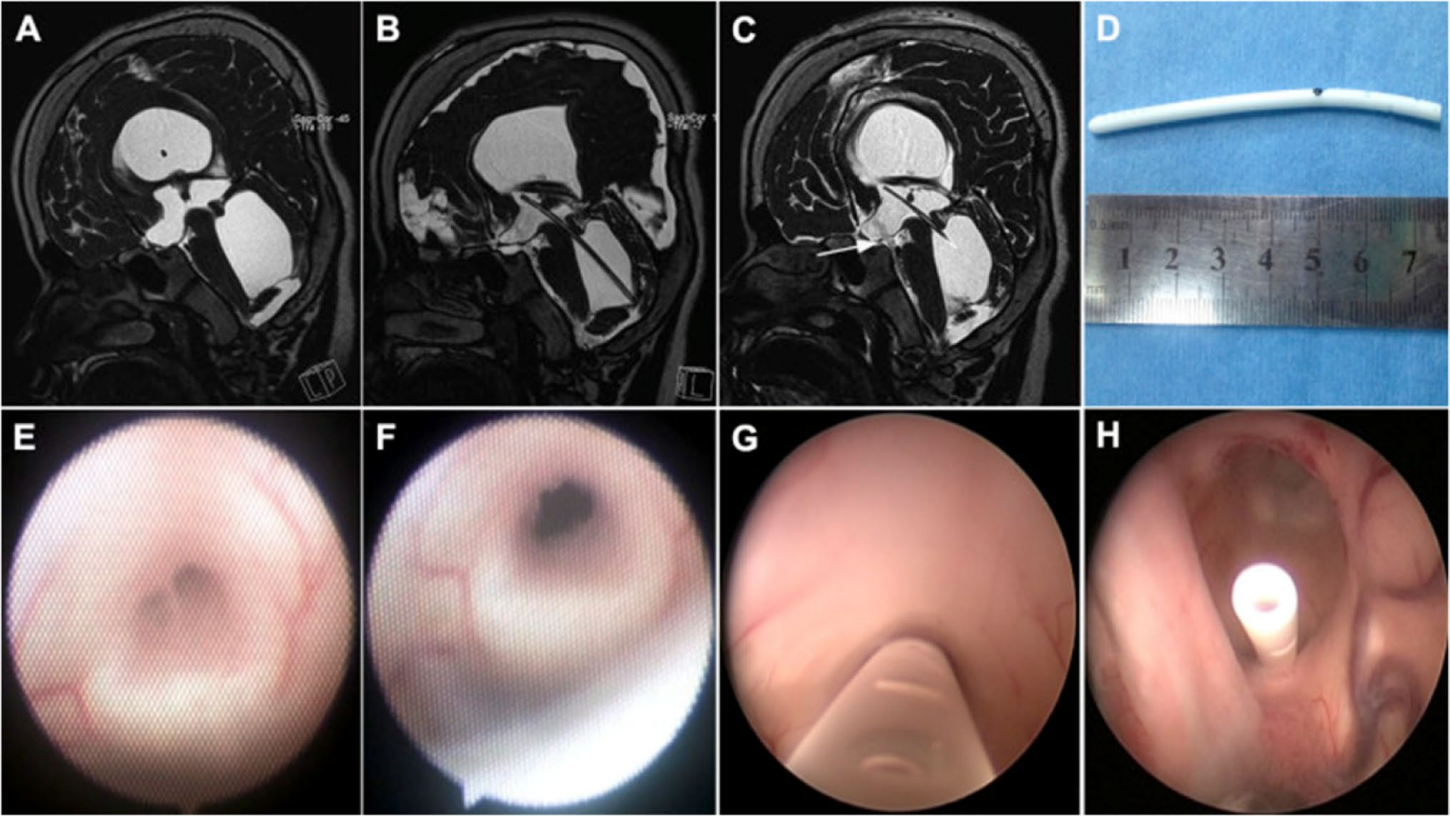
Example of aqueductal stenting (reproduced from Geng et al. [11] with permission). Preoperative sagittal (A) magnetic resonance imaging (MRI) demonstrates the aqueduct obstruction associated the large trapped fourth ventricle. Post-operative MRI scans confirmed the stent in good position (B) and flow void signal (white arrow) through the anterior floor of the third ventricle (C). The tailored aqueduct stent is shown (D). Intraoperative findings with the flexible scope revealed membranous aqueductal stenosis (E), and patency after aqueductoplasty (F). After aqueduct stent placement, the rigid scope was used to check the surface of the catheter to ensure the presence of side hole into the third ventricle (G). Final inspection confirmed that the stent coming from the aqueduct through the foramen of Monro into the lateral ventricle (H)
